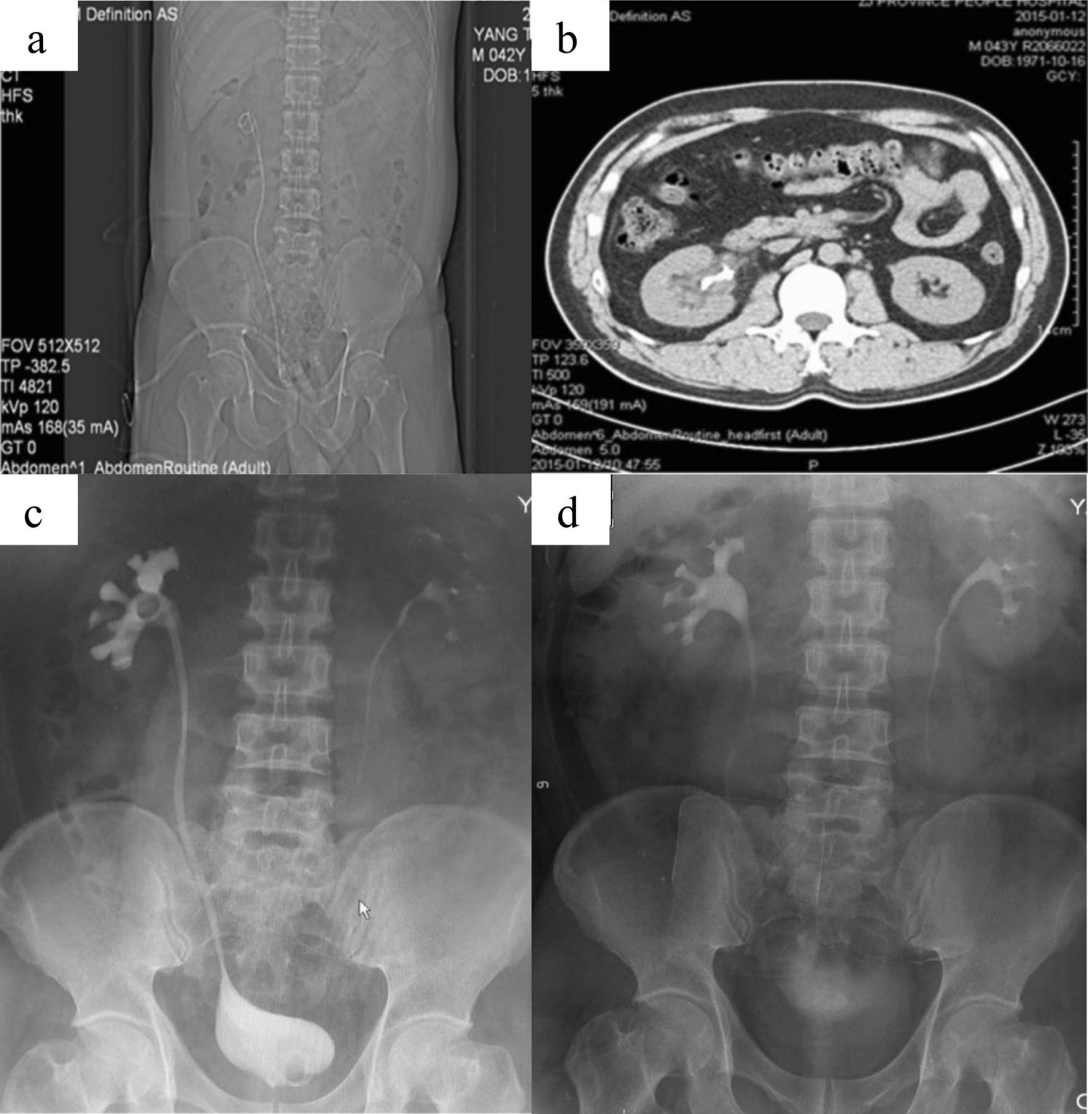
(a) patient nr.2 postoperative KUB; (b) patient nr.2 postoperative 3 months CT, before double J tube removed; (c) patient nr.2 postoperative 3 months IVP with double J tube; (d) patient nr.2 postoperative 12 months IVP.
